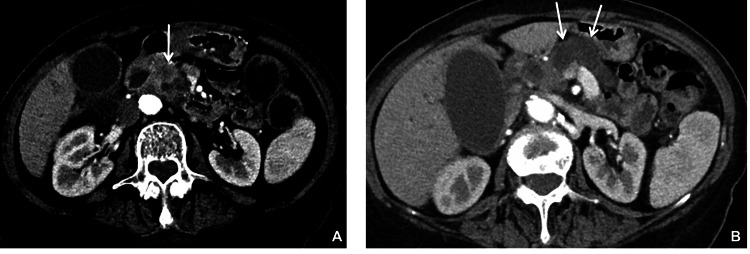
Abdominal contrast medium computed tomography of 2017 (A) Vertical arrow indicates a suspected malignant neoplastic nodule at the pancreas head. (B) The two arrows indicate Wirsung duct ectasia with almost complete atrophy of the pancreatic parenchyma.
